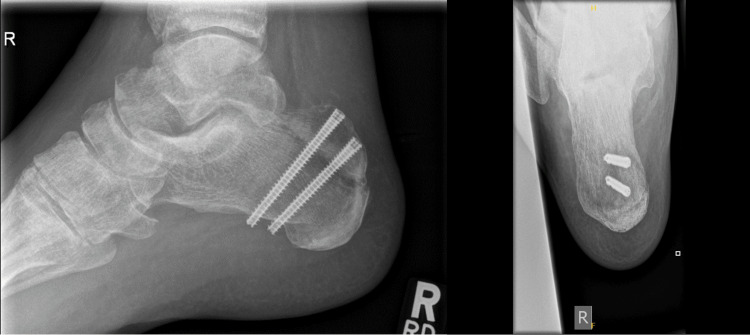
Final plain film radiographs taken twelve weeks post-operatively show an almost complete fracture union
